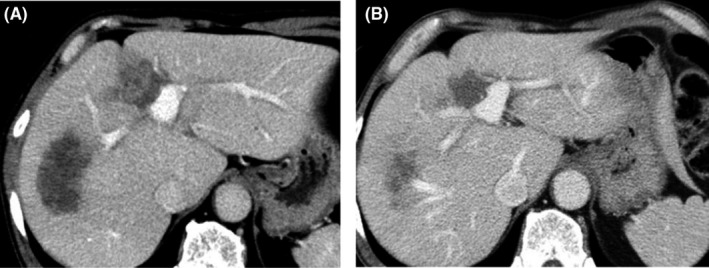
Enhanced computed tomography images after adjuvant chemotherapy. A, Images after eight rounds of adjuvant chemotherapy showing that the liver tumors decreased in size. B, Images after additional chemotherapy showing further reduction in the sizes of the liver tumors and improvement of the invaded umbilical portion
